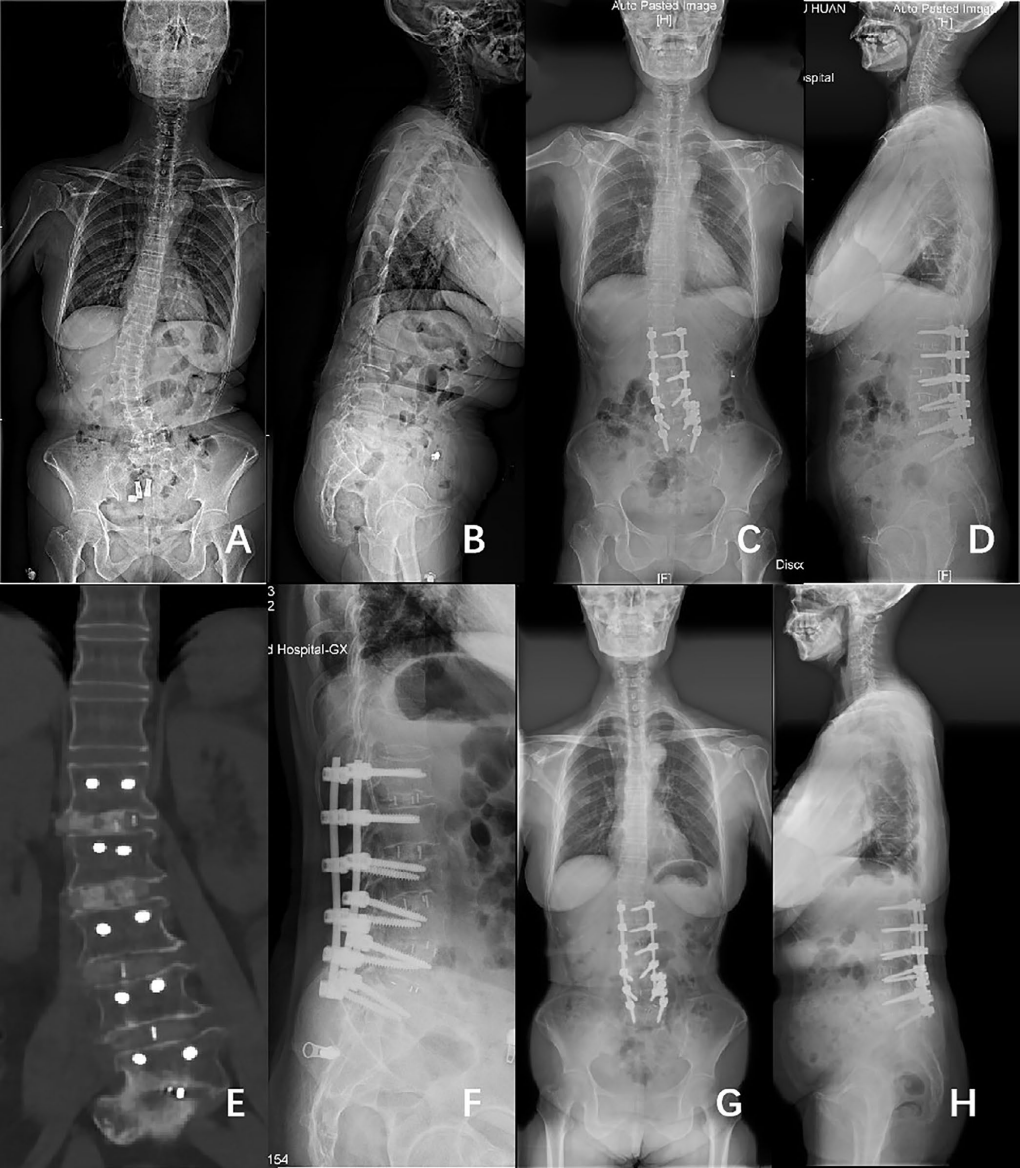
Case example of a 62‐year‐old female with DLS who underwent surgical correction consisting of L1–L5 OLIF with posterior instrumentation. (A and B) Full‐length standing posteroanterior and lateral radiographs before operation (Cobb 32.0°, CBD 19.84 mm, PI 58.3°, PT 36.3°, SS 22.1°, TK 2.9°, LL 1.5°, SVA 145.25 mm). (C and D) Posteroanterior and lateral standing radiographs 1 year after operation (Cobb 8.0°, CBD 13.51 mm, PI 58.3°, PT 35.8°, SS 22.8°, TK 15.5°, LL 26.8°, SVA 48.46 mm). (E) CT scan 1 year after surgery showed bone fusion at L1–L5. (F) Cage subsidence was observed 1 year after surgery. (G and H) Posteroanterior and lateral standing radiographs 2 years after operation (Cobb 8.6°, CBD 15.83 mm, PI 52.8°, PT 35.7°, SS 17.3°, TK 6.5°, LL 17.5°, SVA 51.84 mm).
